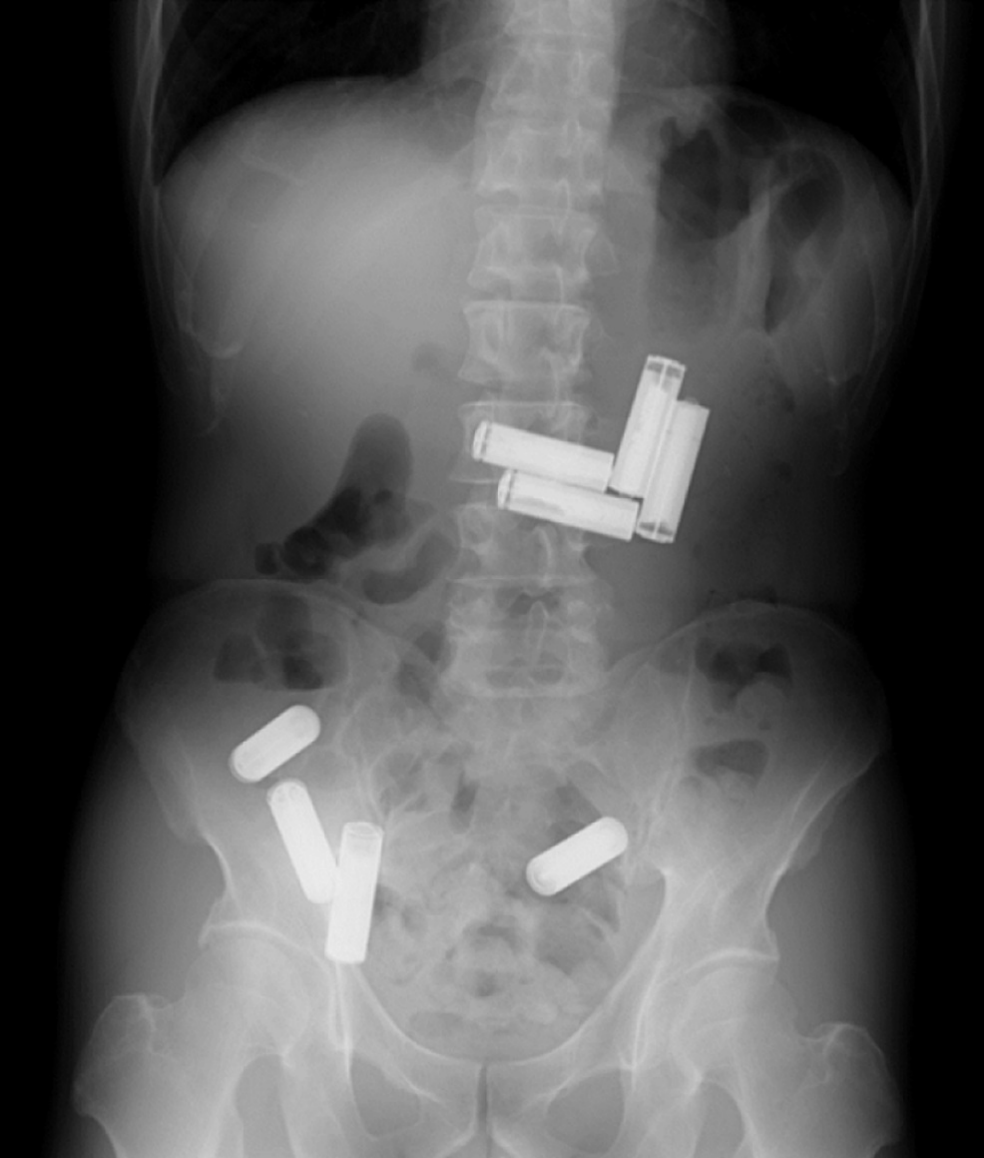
Plain abdominal x-ray showing eight cylindrical batteries in the left upper and lower abdomen.
